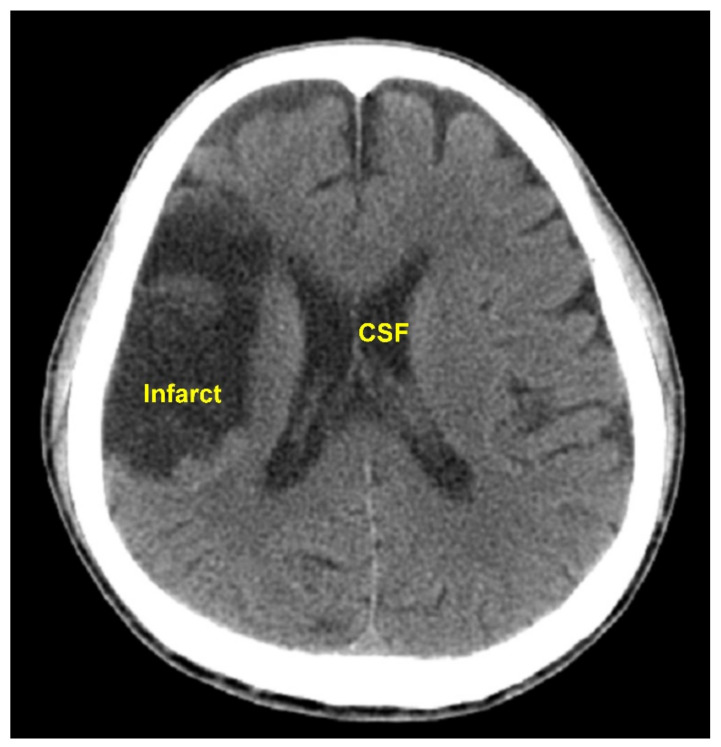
This CT image shows similar intensities of the CST and the infarct region. The CST could be falsely detected as infarcted if not eliminated before the infarct detection.
